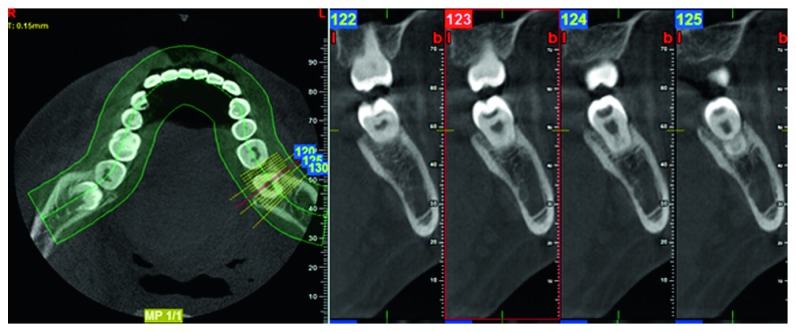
Sagittal slices of the PEIR-affected tooth (permanent mandibular left second molar) reconstructed from the second CBCT when the patient was 14 years, 9 months old. The PEIR location was reconfirmed to be just under the dentino-enamel junction and not connected to the pulp chamber or oral environment. The distance between the midfloor of the lesion and the pulpal area is 2.00 mm. The PEIR dimension is 2.30 mm occluso-gingival depth, 4.00 mm bucco-lingual width, and 3.40 mm mesio-distal length.
